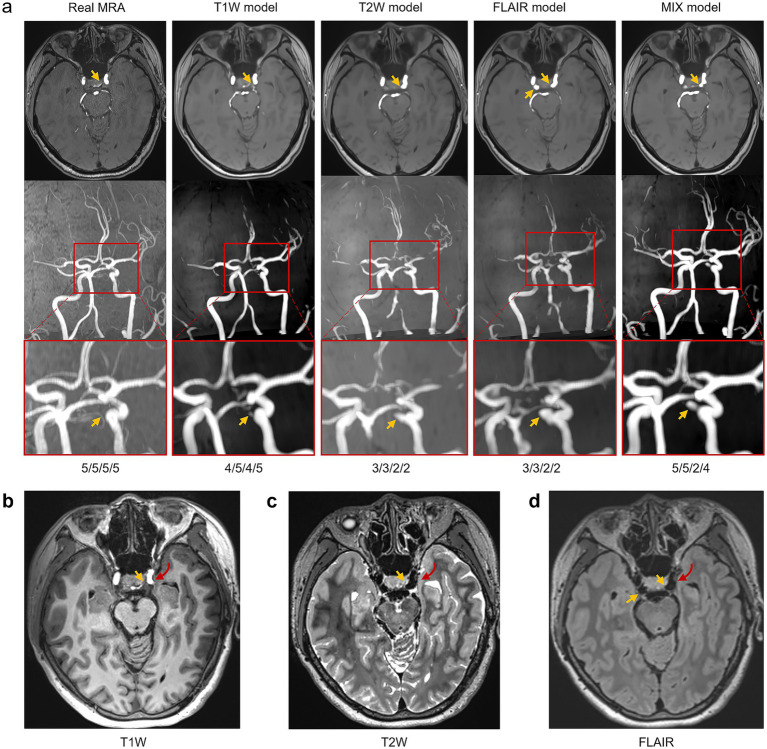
A representative case of cavernous sinus cavity-mediated false-positive aneurysm in synthetic MRA. (a) A 56-year-old female with real MRA demonstrating no abnormalities. Synthetic MRA images from T2W, FLAIR, and MIX model depicted a false-positive aneurysm at the left internal carotid artery C4 segment, absent in T1W synthetic MRA (yellow arrow). (b) The internal carotid artery demonstrated hyperintensity on T1W source images (red curved arrow), while the location of false-positive aneurysm was hypointense cavernous sinus cavity (yellow arrow). (c,d) T2W and FLAIR source images showed flow void signal in both internal carotid artery (red curved arrow) and cavernous sinus cavity (yellow arrow). The red box indicates the magnified image, n/n/n/n means overall image quality/vascular edge sharpness/venous contamination/diagnostic confidence scores. MRA = magnetic resonance angiography, T1W = T1-weighted; T2W = T2-weighted, FLAIR = fluid attenuated inversion recovery.
